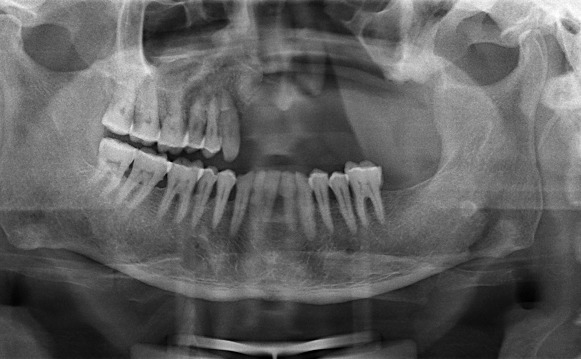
Panaromic radiograph showing multiple missing teeth and features suggestive of left maxillectomy
